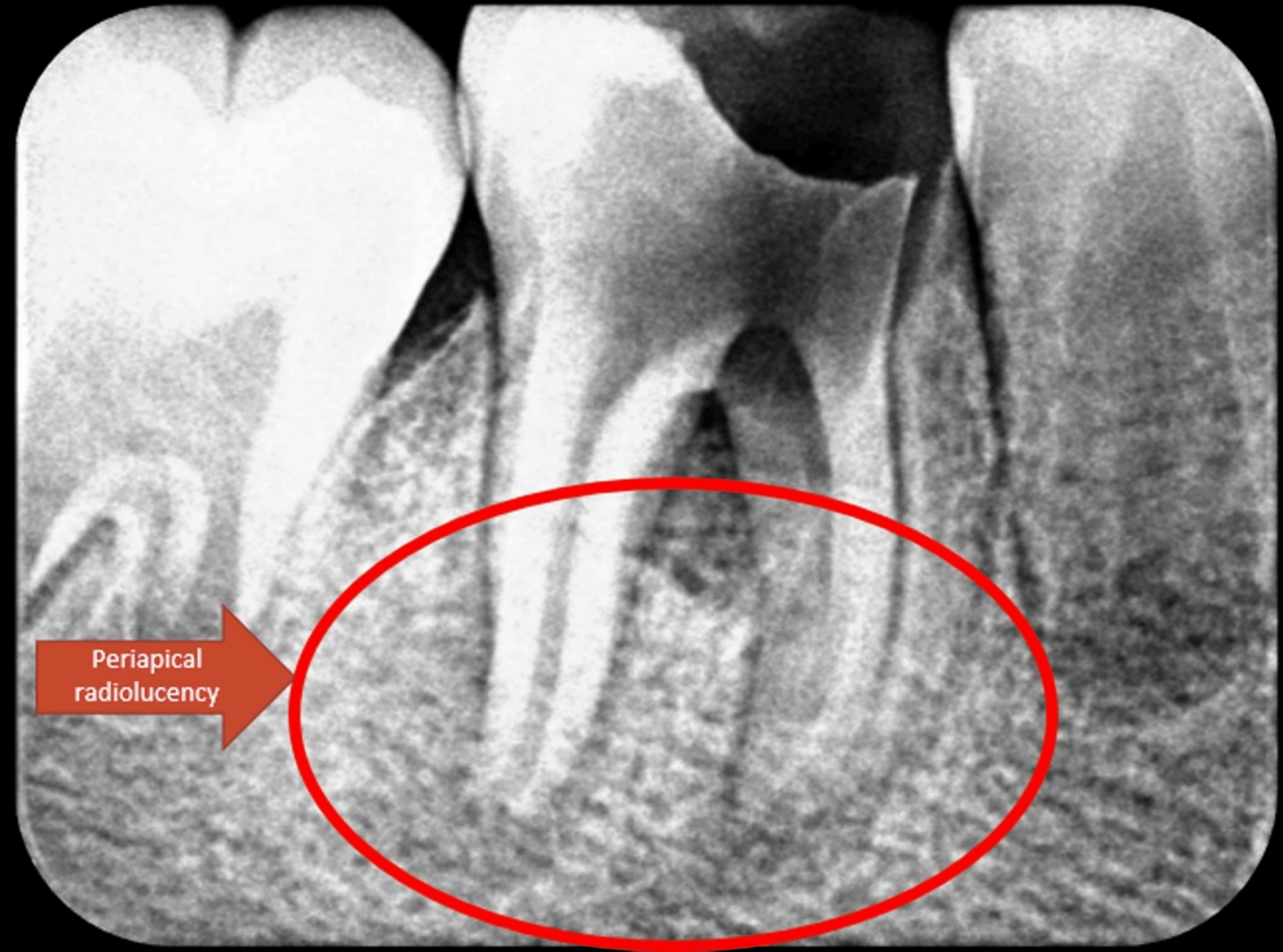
Pre-treatment intra-oral periapical (IOPA) radiograph with respect to 46 showing occlusal radiolucency involving enamel, dentine and pulp, periodontal ligament widening, furcation involvement with inter-radicular and periapical radiolucency
